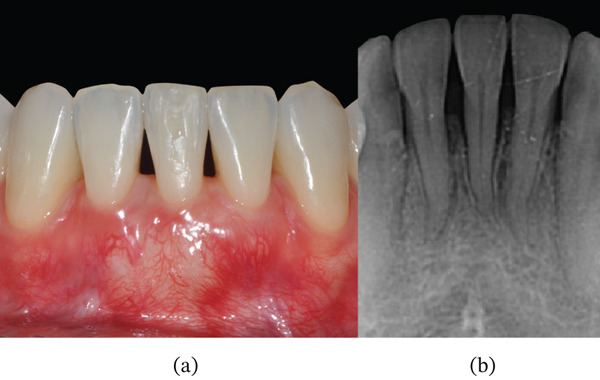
Three‐year follow‐up: (a) clinical and (b) radiographic view.
